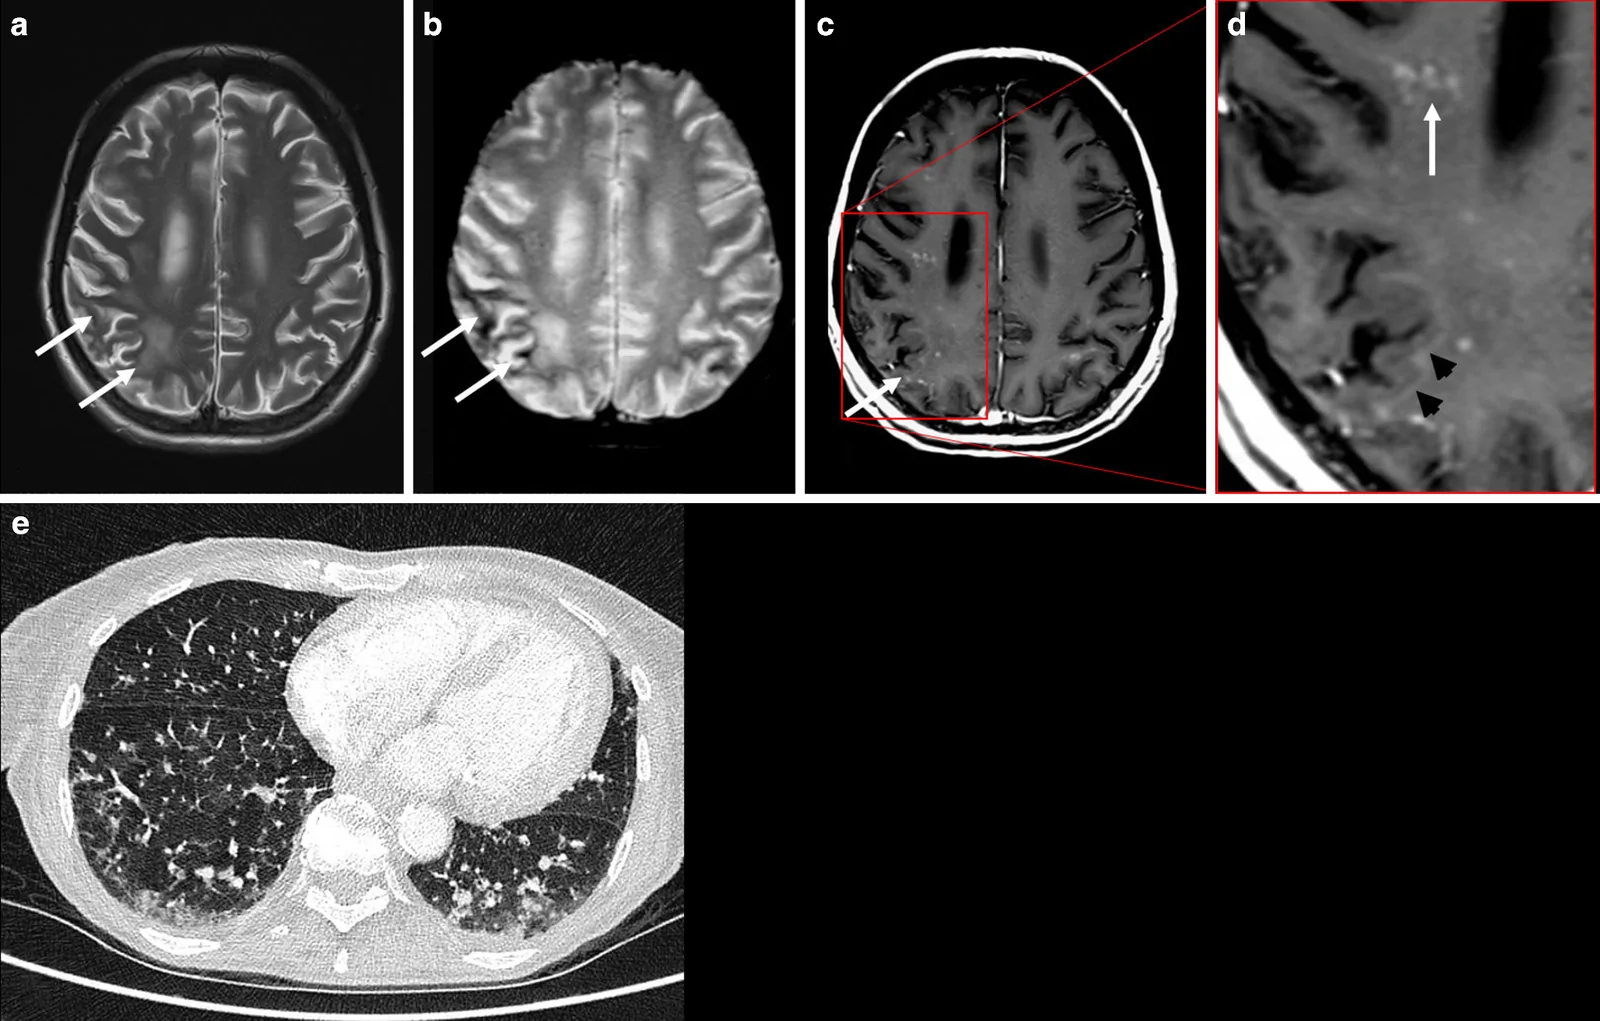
Lymphomatoid granulomatosis. a–d T2-hyperintense subcortical parietal right-sided lesion (a arrow) involving also the U‑fibers with inhomogeneous signal loss on T2*-weighted images (WI; b arrow) and multifocal punctuate (c, d arrow) as well as bandlike subcortical enhancement (d arrowhead), note further left-sided parietal lesion; e thoracic CT disclosing multiple granulomatous pulmonary lesions
